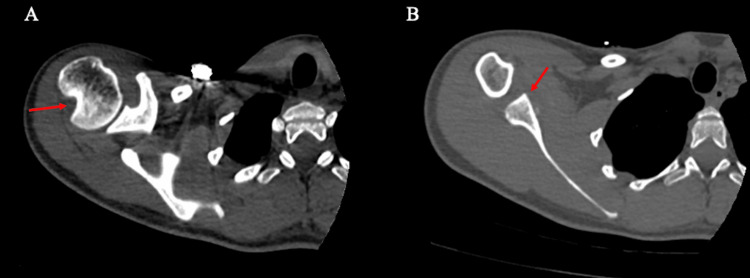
Axial CT scan (case 2) (A) large Hill-Sachs lesion and (B) glenoid bone loss CT: computed tomography
